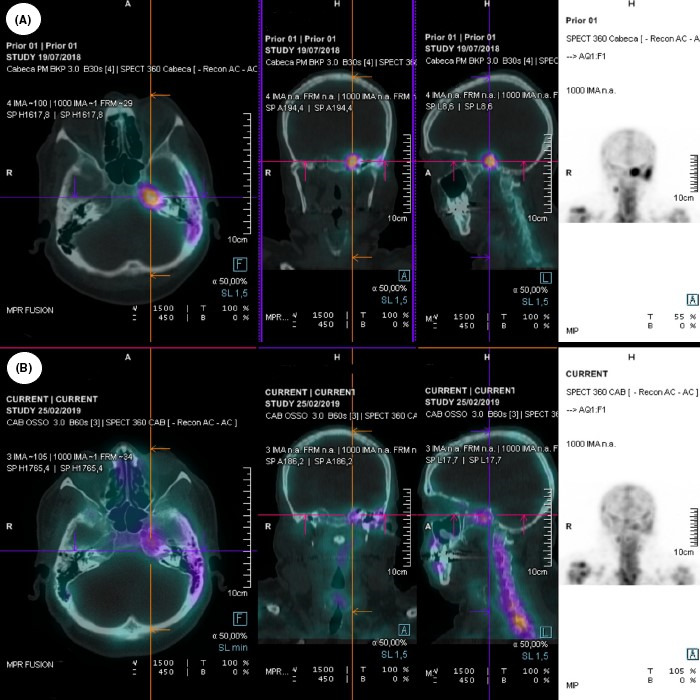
Technetium scintigraphy. A, First technetium scintigraphy showing hypercapture in the left mastoid and petrous apex suggesting inflammatory process. B, Interval technetium scintigraphy at the follow‐up showing resolution of the inflammatory process in the left temporal bone
